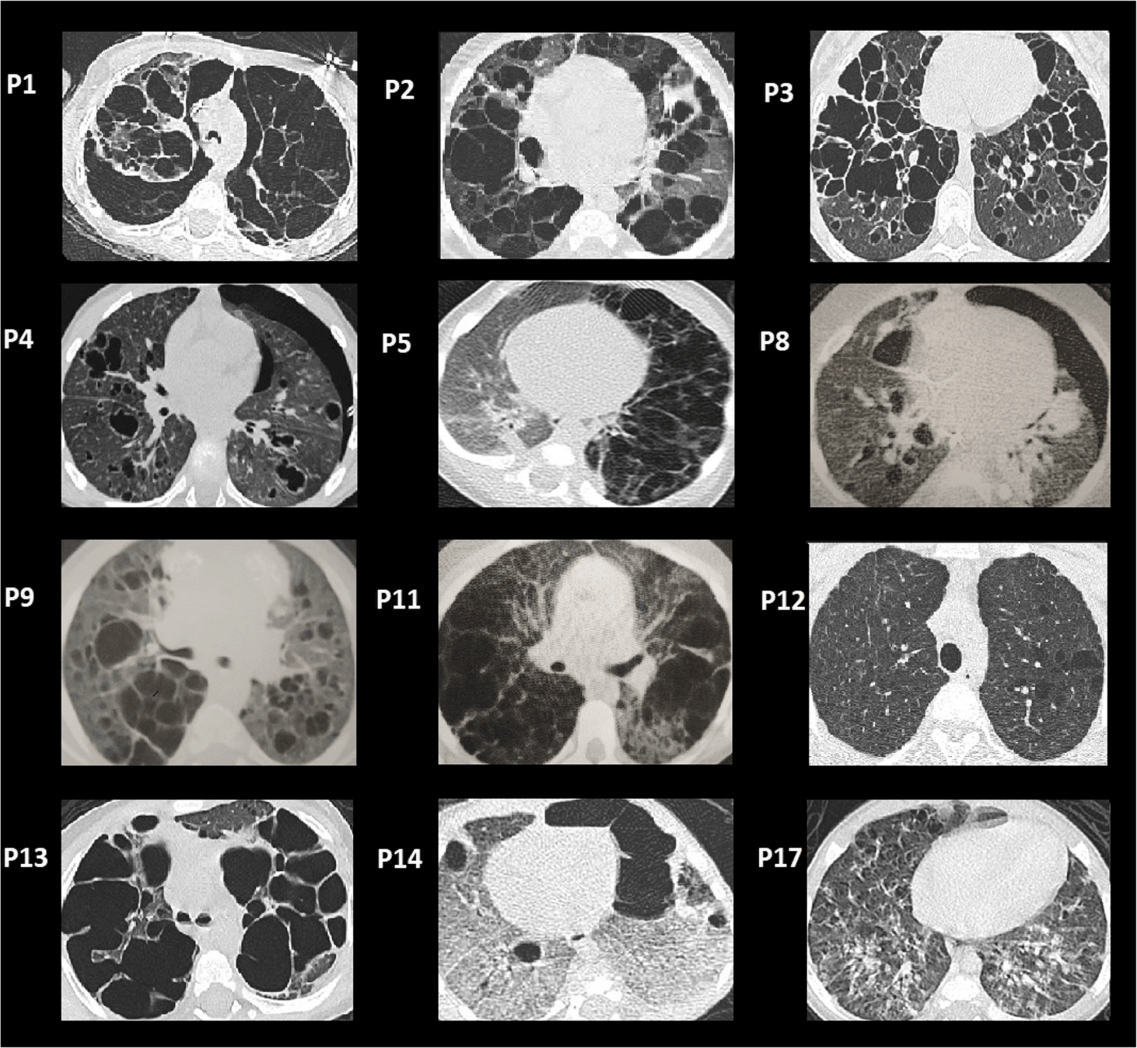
Chest CT scan images (lung window). Initial images of PLCH for 12 of 17 patients. PLCH: Pulmonary Langerhans cell histiocytosis
